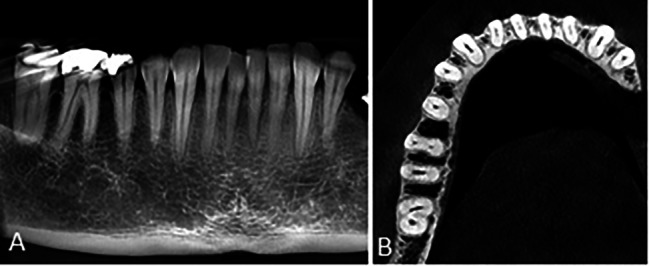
Mandibular second molar tooth with (A) type 4 morphology on the panoramic-like image and (B) C-shaped (C2) pattern on the axial CBCT section
